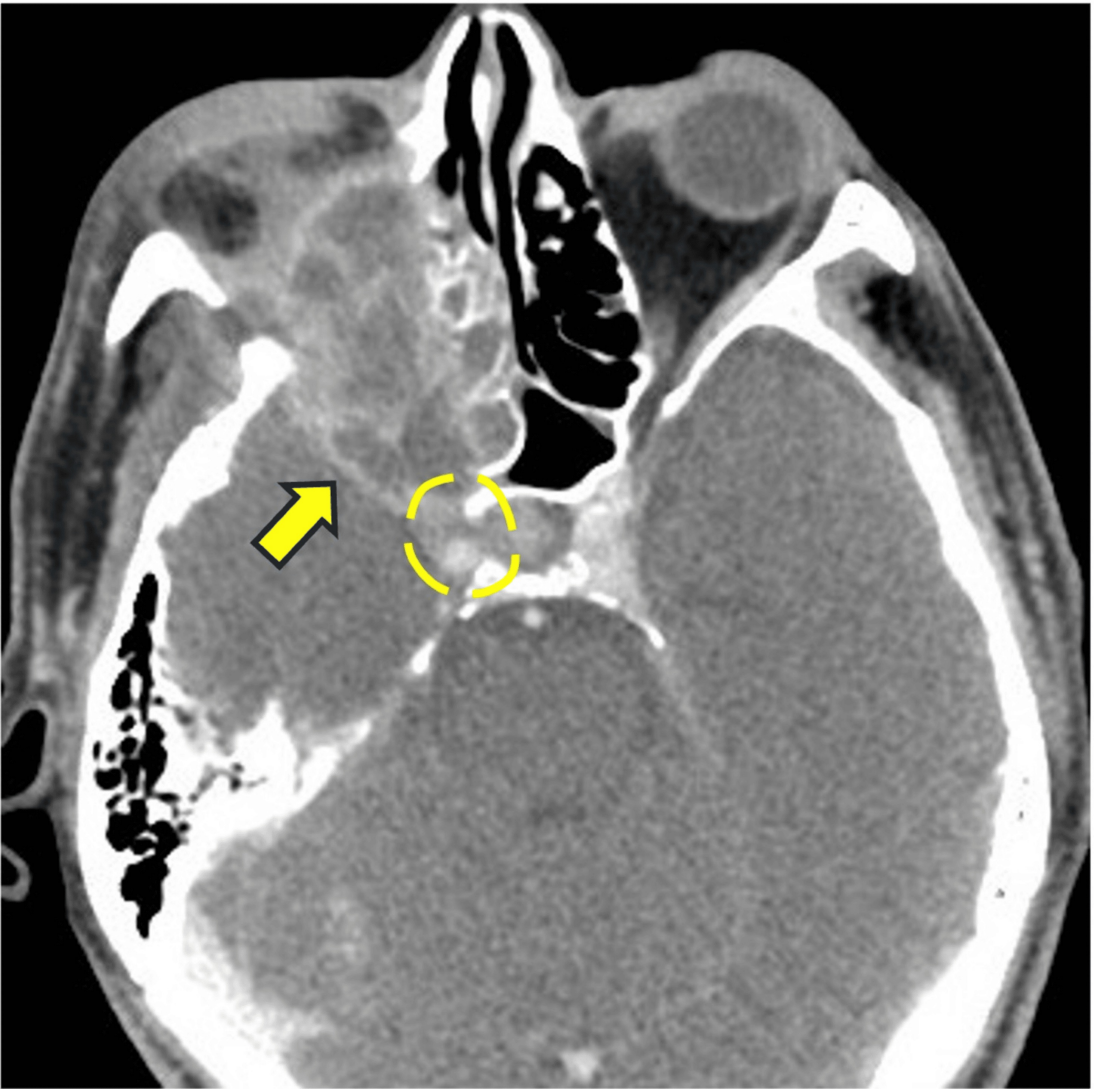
Contrast-enhanced CT of the sinus image (axial section) The lesion extended to the right orbital apex. Inflammation was also noted extending to the dura mater, with infiltration into the cavernous sinus.
